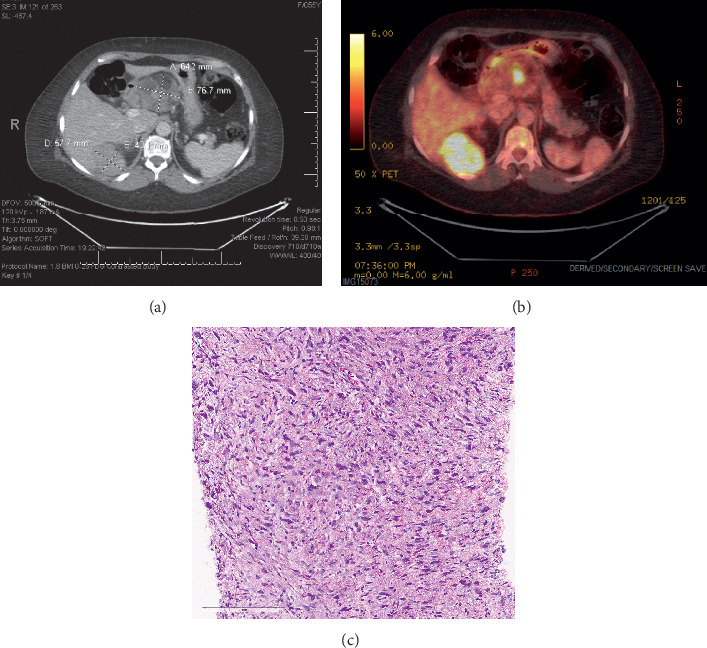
CT (a) versus PET (b) from a patient where the initial biopsy, done prior to the PET directed at the mass adjacent to the pancreas, showed WDLPS. The PET clearly shows only a small area within this mass to have FDG activity. Repeat biopsy from the FDG avid right perinephric mass demonstrated cellular nonlipogenic component with pleomorphic and atypical spindle cells and mitoses consistent with DDLPS (c).
